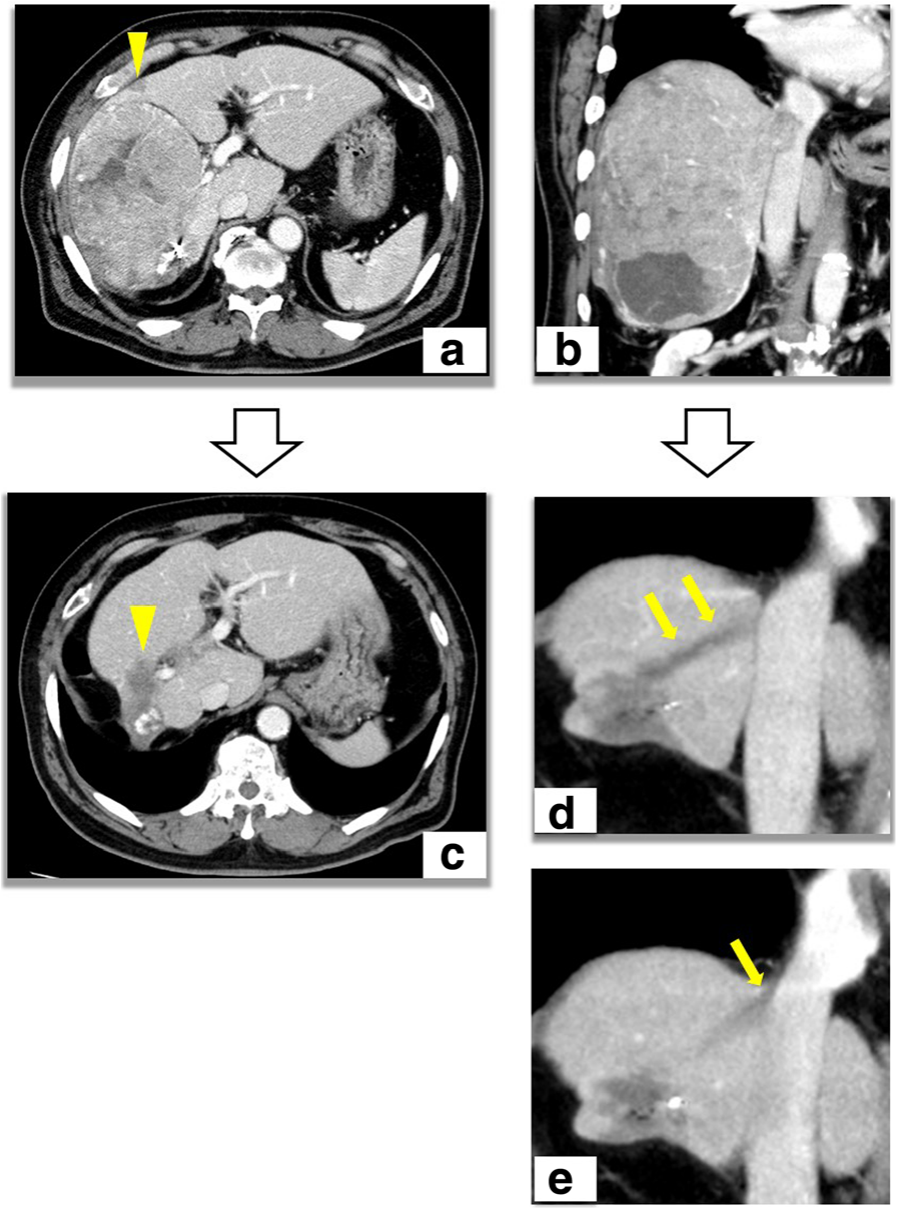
CT of the abdomen. a HCC with a maximum diameter of 16.0 cm in the right lobe detected before HAIC; intrahepatic metastasis is marked by the arrowhead. b HVTT extending from the RHV to the IVC prior to HAIC. c The size of the main HCC markedly decreased (*arrowhead*), with no obvious intrahepatic metastases after 13 courses of HAIC. d-e After 13 courses of HAIC, the advanced part of the HVTT went down to the root of the RHV (*arrows*)
